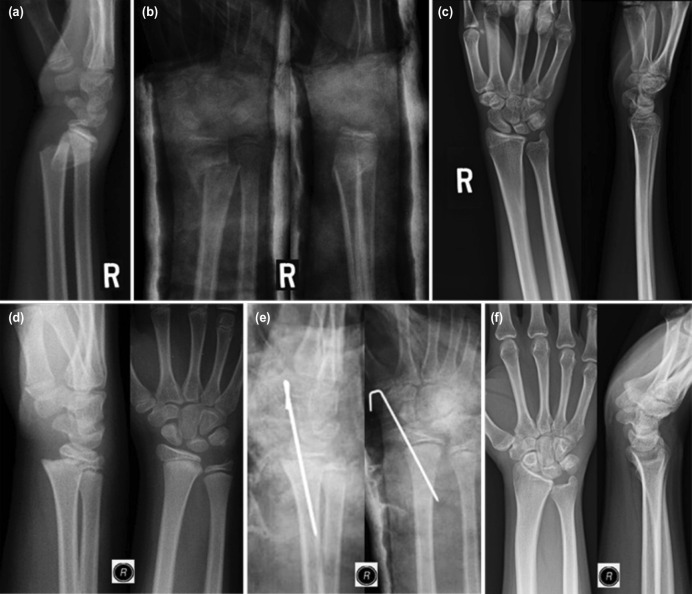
(a) Photos showing the pre-reduction lateral radiograph of a metaphyseal fracture of the distal right radius with dorsal angulation about 45° in a 13-year-old boy, (b) immediate reduction of the fracture followed by a cast application improved the dorsal angulation on lateral radiograph and showed good bone contact on AP view, (c) good remodeling and bone union as seen at skeletal maturity, (d) distal physeal fracture of the right radius on a different child who was a 12-year-old boy with dorsal translation and dorsal tilt of the epiphysis, (e) closed reduction and percutaneous smooth K wire corrected the displacement and secured the reduction, (f) and finally AP and lateral radiographs at skeletal maturity revealed complete healing of the fracture without evidence of physeal arrest.
